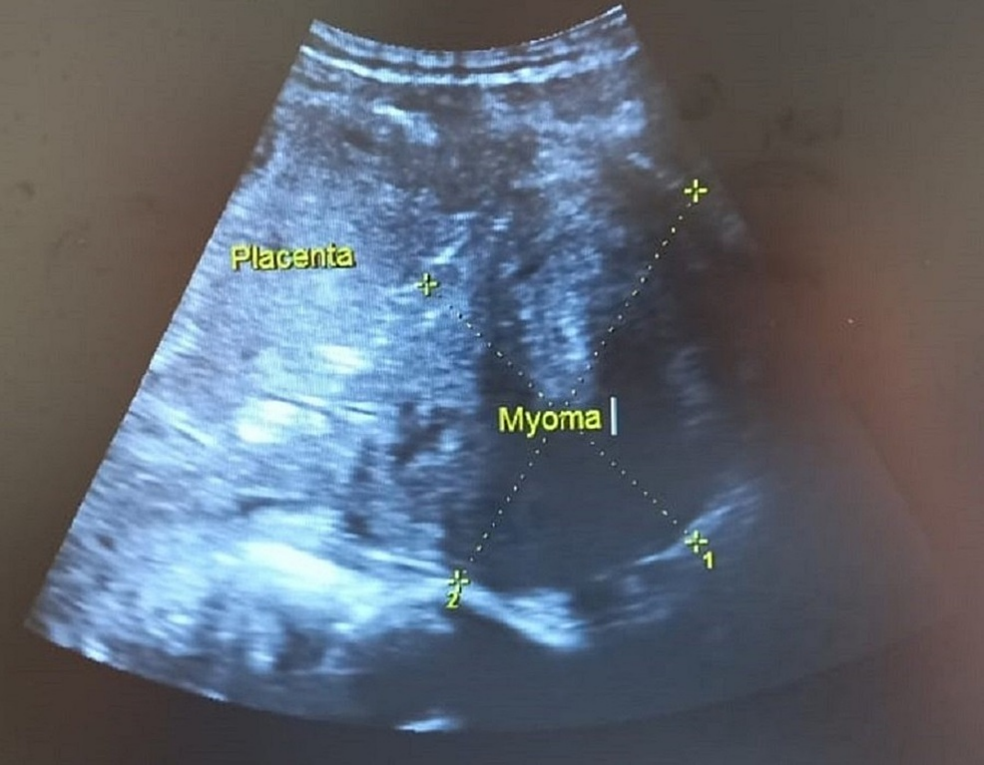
Abdominal ultrasound image showing central placenta previa and a cervical fibroid or myoma of size 10 cm x 8 cm
